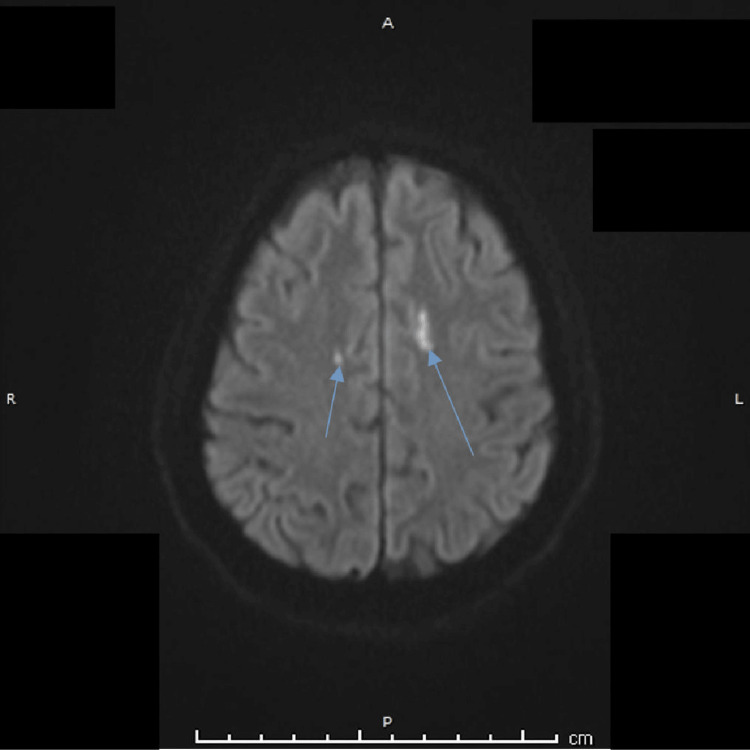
Axial diffusion-weighted imaging (DWI) obtained eight days after presentation demonstrating a bilateral hyperintense signal within the high frontal regions (arrows), consistent with diffusion restriction in keeping with bilateral anterior cerebral artery (ACA) territory infarctions.
